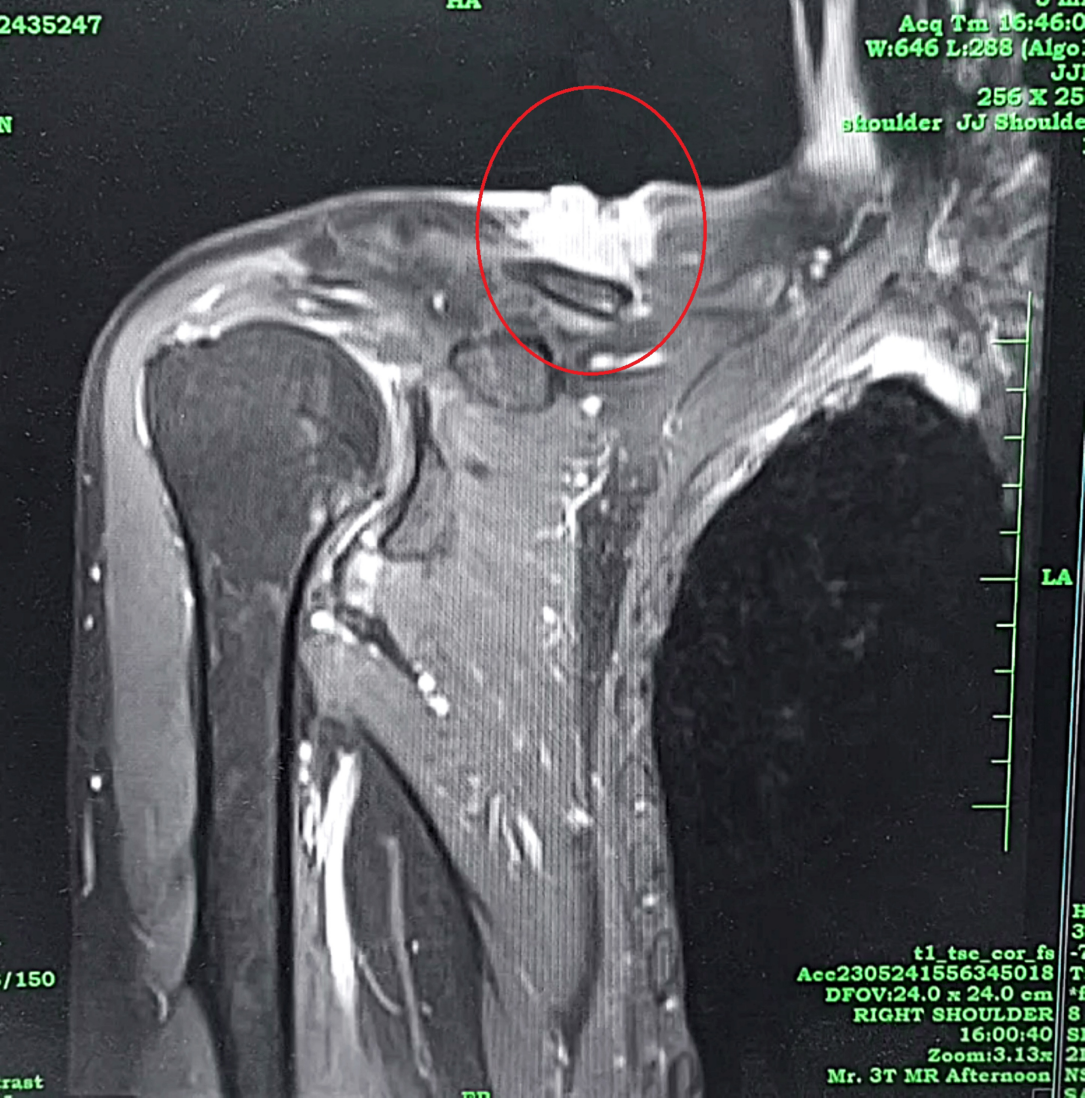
MRI STIR image showing a hyper-intense signal at the site of lesion STIR: short tau inversion recovery
